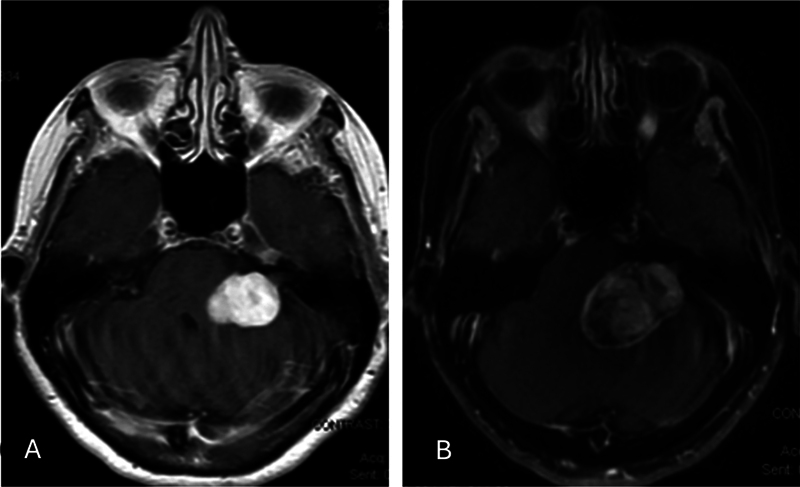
Initial contrast MRI of brain showing a homogeneously enhancing mass of the left cerebellopontine angle (CPA) in axial view (A). Follow-up contrast MRI of brain 3 weeks after the initial MRI demonstrating radiographic progression from a homogeneous enhanced lesion to heterogeneous enhanced lesion (B).
